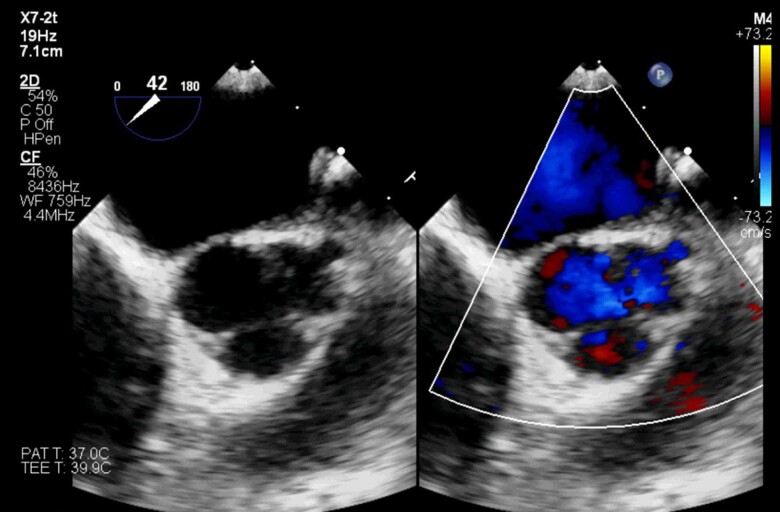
Transoesophageal echocardiogram demonstrating unobstructed left coronary artery flow post repair.
